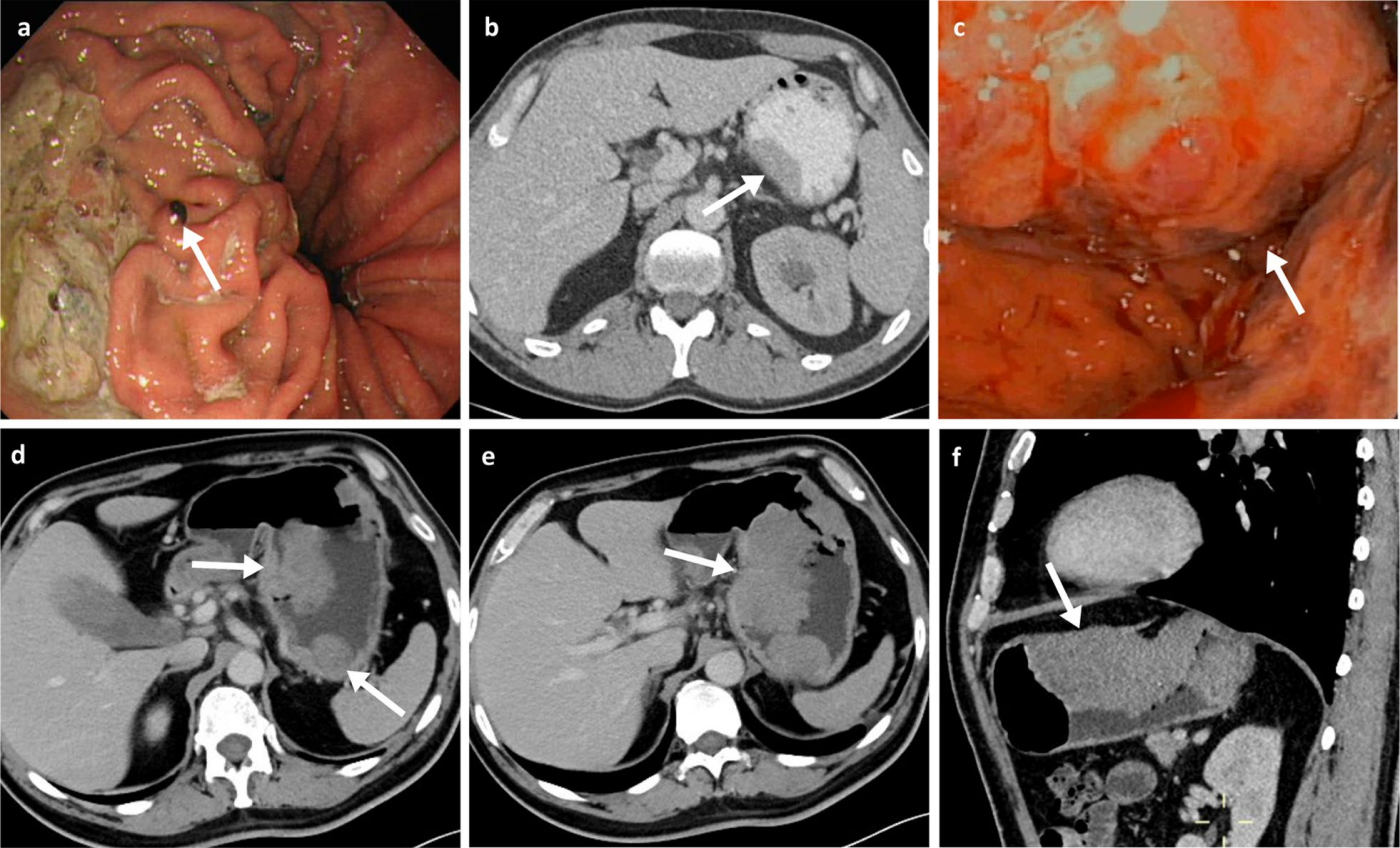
Gastric melanoma metastases. a Endoscopy of a 69-year-old female patient with a melanotic nodule in the proximal gastric body (arrow). b Oral and intravenous portal phase contrast-enhanced CT axial of a 42-year-old male patient with a sessile soft-tissue melanoma metastasis associated with the lesser curvature (arrow). c–f Endoscopy and portal phase contrast-enhanced CT axial and sagittal of a 51-year-old male patient demonstrating a large melanoma metastasis within the body of the stomach with resulting luminal narrowing (arrows)
